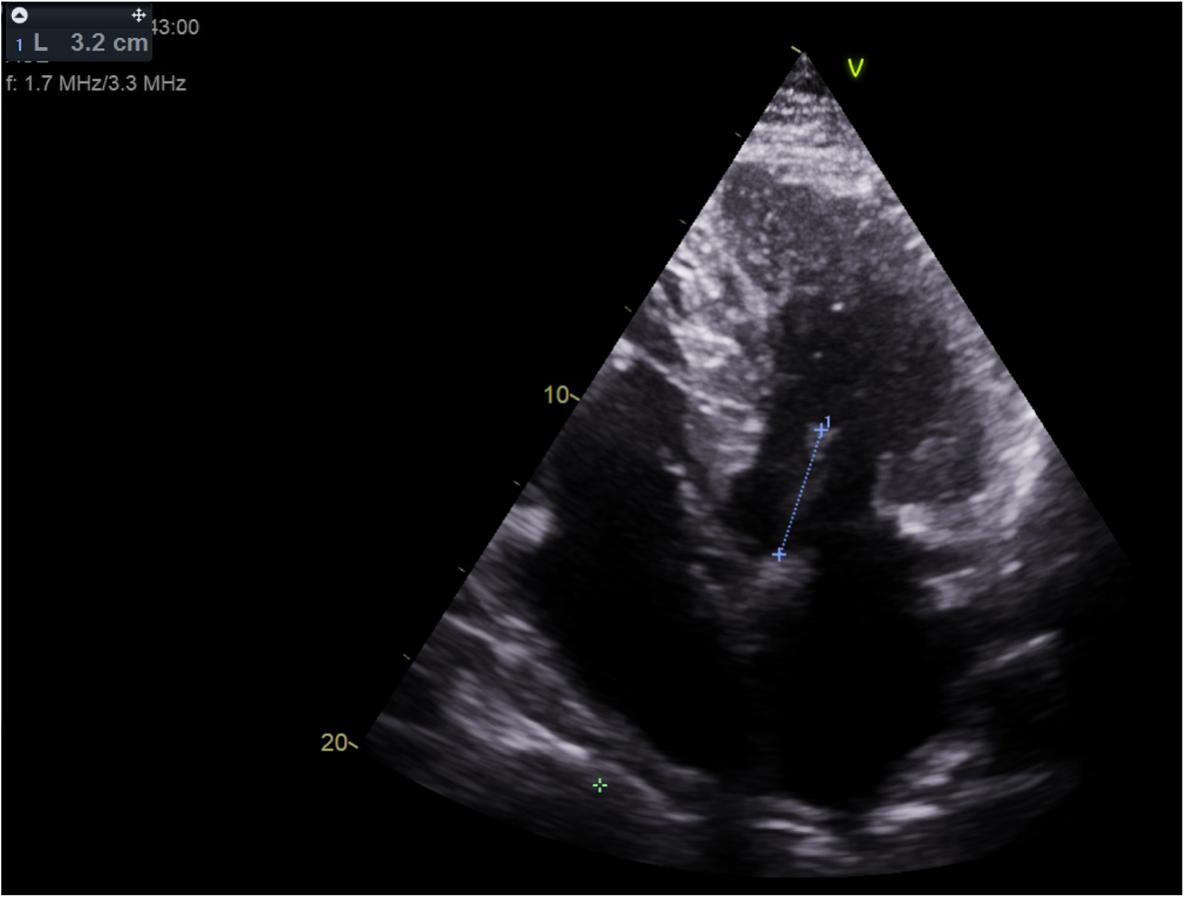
Anterior mitral valve leaflet length, apical four chamber view (4CH)
